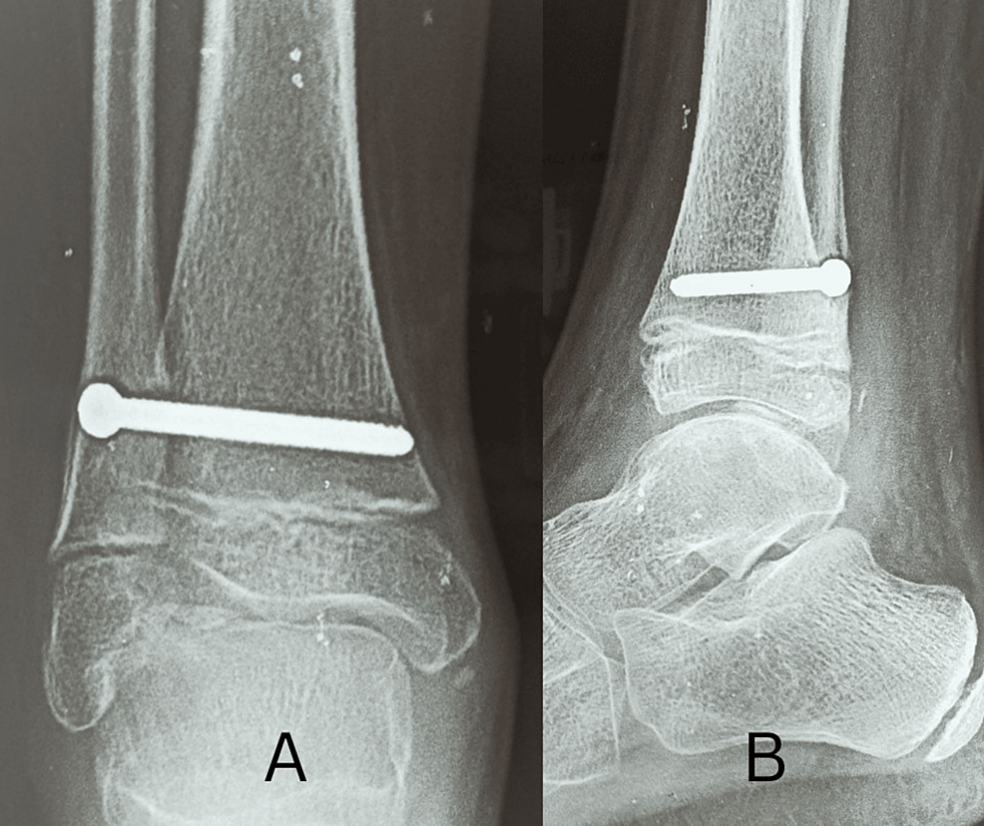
Six months follow-up AP (A) and lateral (B) radiographs showing good reconstruction of mortise
